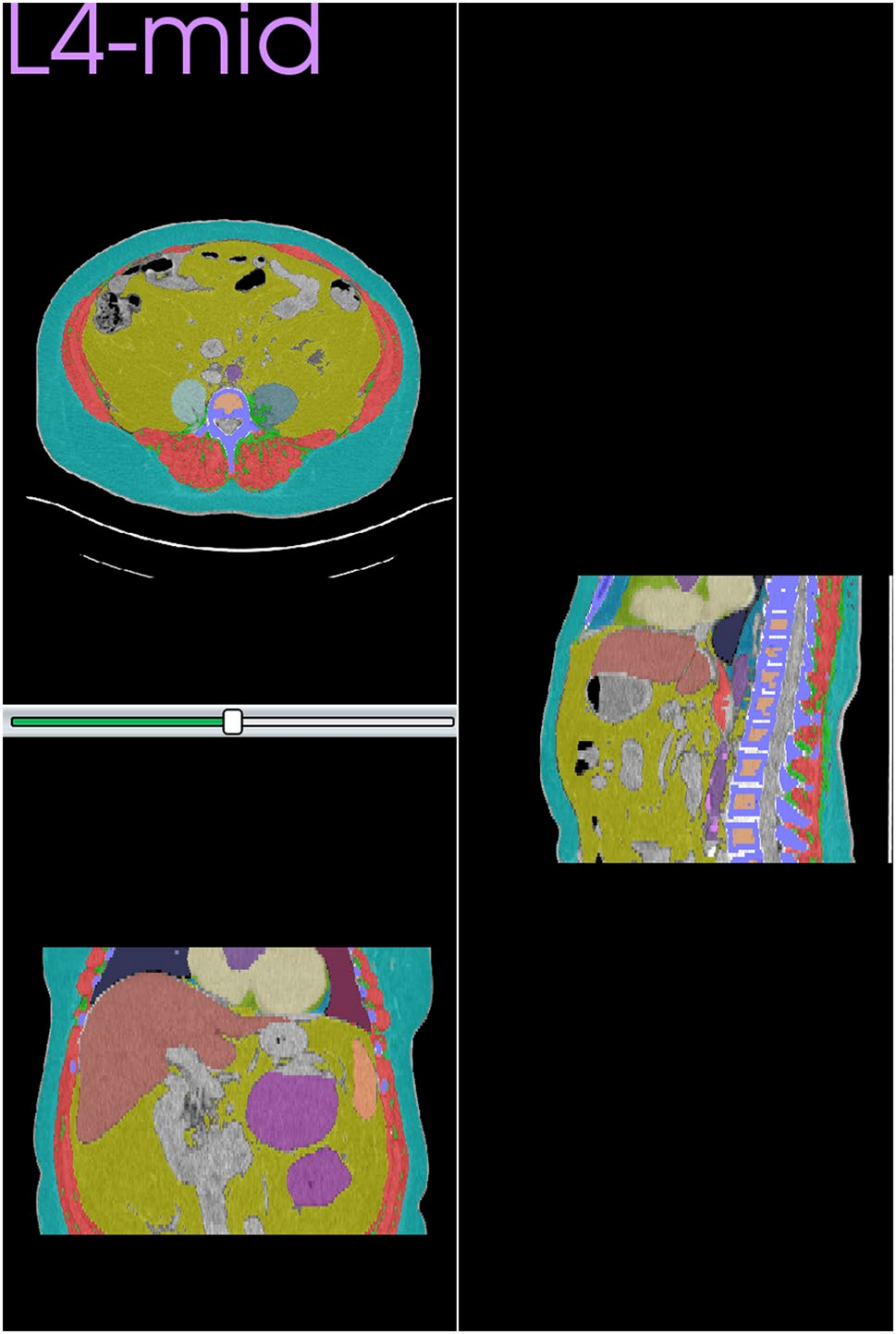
Axial CT scan image at L4 mid with automated body composition in a 53-year-old woman with ACC.
